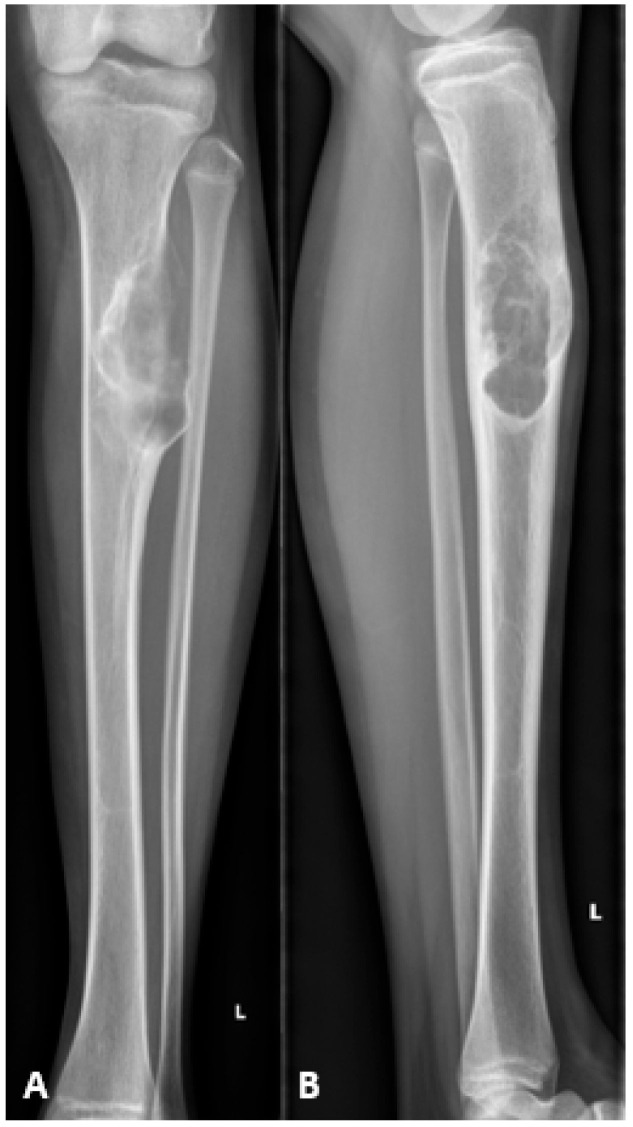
Preoperative radiographs showing an osteofibrous dysplasia-like adamantinoma (OFD/LA) in the left tibial diaphysis. (A) Anteroposterior view. (B) Lateral view.
